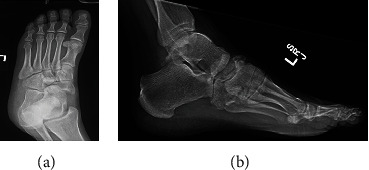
(a) Initial AP X-ray of the left foot demonstrating subtle talonavicular dislocation, read as negative by radiology. (b) Initial lateral X-ray of the left foot demonstrating subtle talonavicular dislocation, read as negative by radiology.
